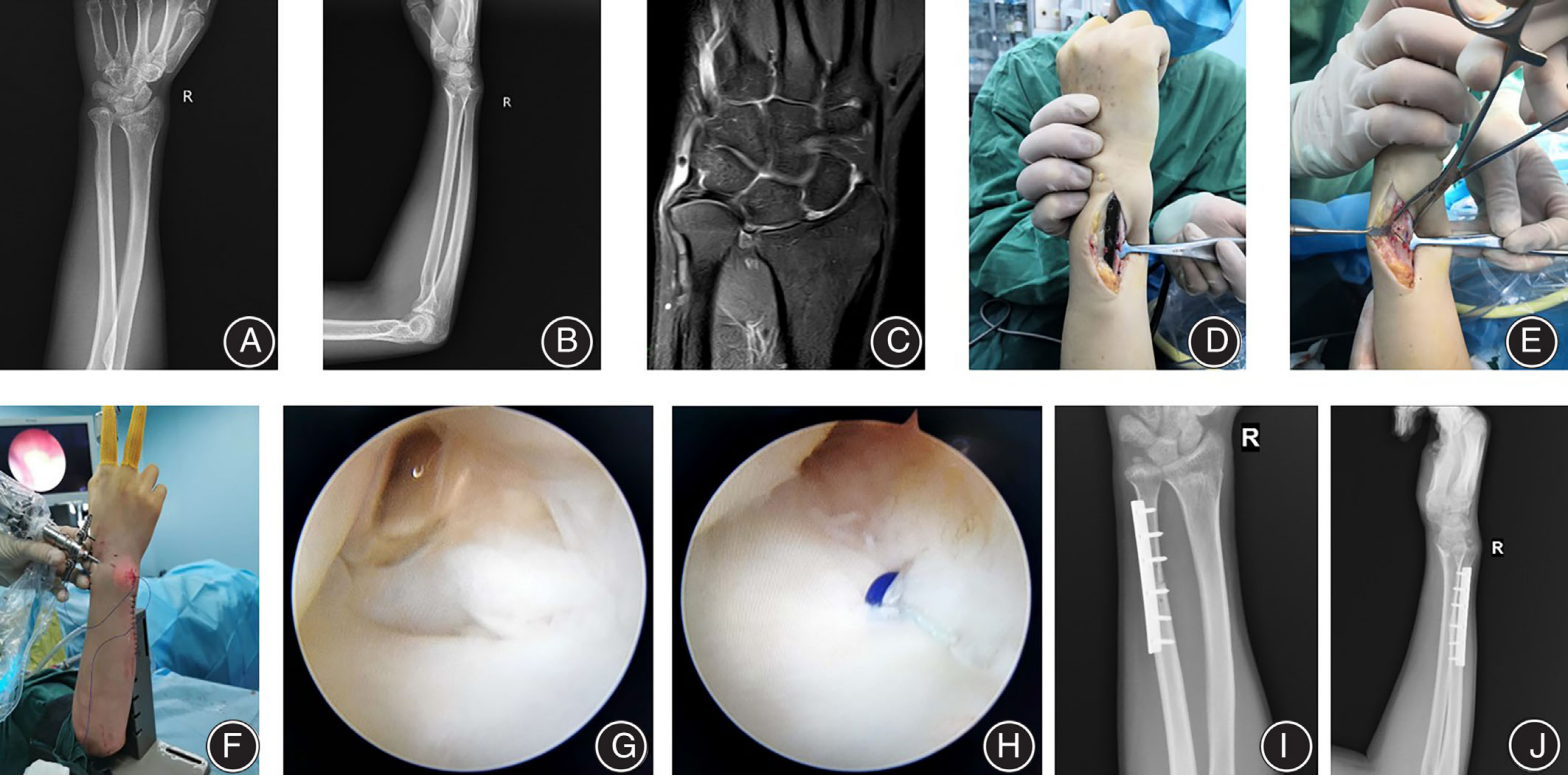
Typical case 1. Female, 36 years old, pain and limited mobility for 8 months due to long‐term activity in the right wrist joint, diagnosed with right wrist UIS, TFCC injury. Treated by wrist arthroscopy combined with oblique USO. (A, B) Preoperative anterolateral X‐ray films showed positive ulna variation; (C) Preoperative MRI showed positive ulnar variation combined with TFCC injury; (D, E) Intraoperative oblique USO. (F) Intraoperative arthroscopic exploration of the wrist; (G, H) Intraoperative suture of TFCC; (I, J) Postoperative X‐rays showed that the ulnar osteotomy was well‐aligned, and the ulnar positive variation had recovered
